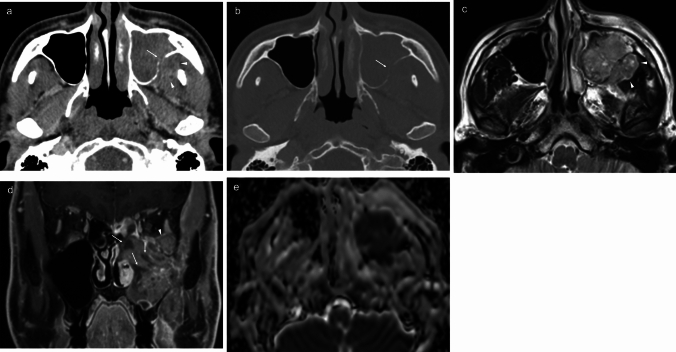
Sinonasal nuclear protein in testis carcinoma (case 5). Contrast-enhanced CT axial image (a) and bone algorithm CT axial image (b) shows a tumor with heterogeneous moderate enhancement in the left maxillary sinus, with destructive changes in the posterior wall of the left maxillary sinus (arrows) and tumor extension into the left retroantral fat pad (arrowhead). T2-weighted axial image (c) shows high signal intensity lesion with tumor extension to the left retroantral fat pad (arrowhead). Post-contrast T1 coronal image (d) shows heterogeneous moderate enhancement of the lesion, with an internal necrotic area (arrows) and intraorbital extension (arrowhead). ADC map (e) demonstrates a value of 0.84 × 10⁻^3^ mm^2^/s in the solid portion of the tumor
